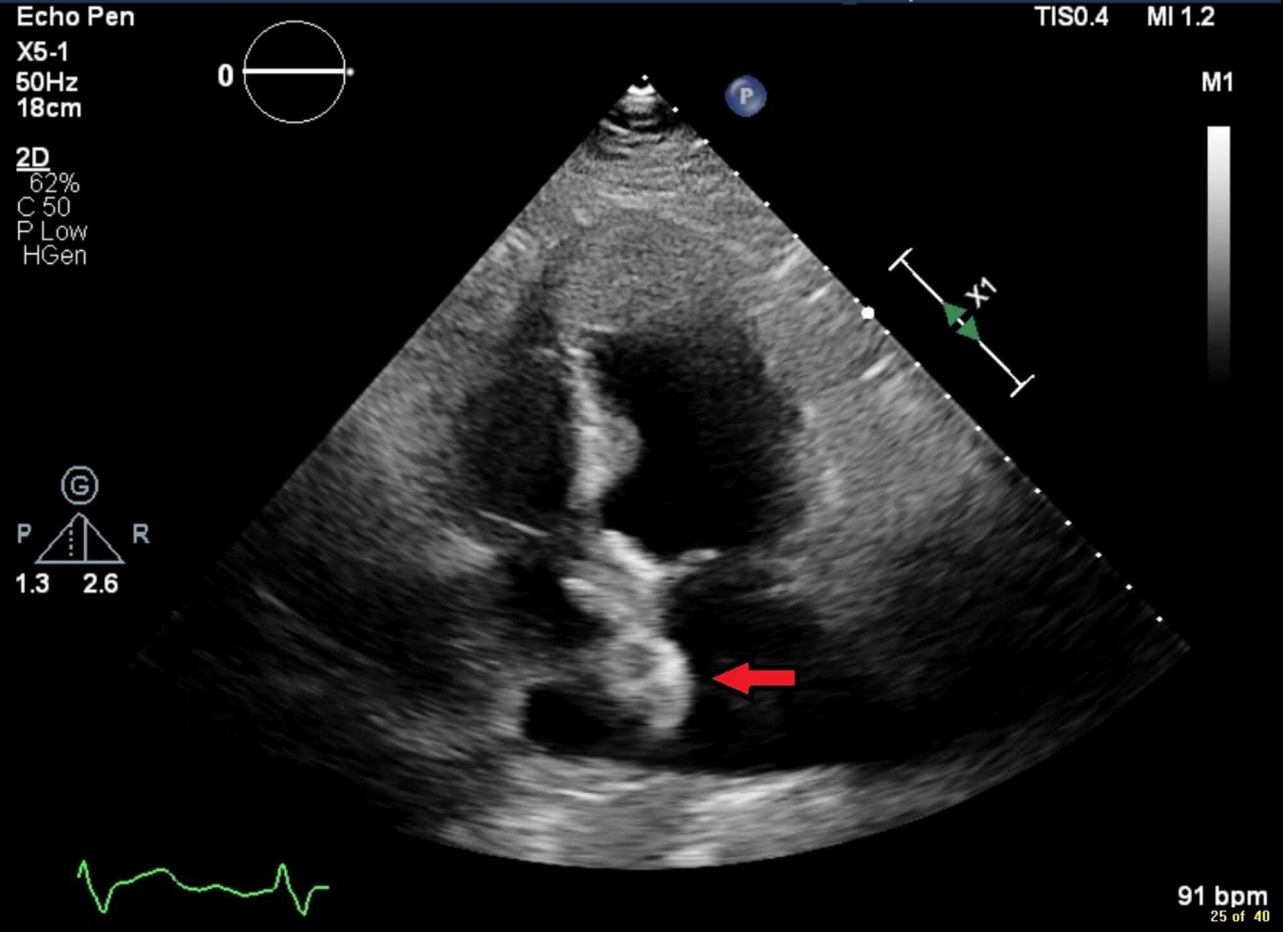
A 25-mm Amplatzer PFO occluder device visualized on a TTE (red arrow). PFO, patent foramen ovale; TTE, transthoracic echocardiogram.
